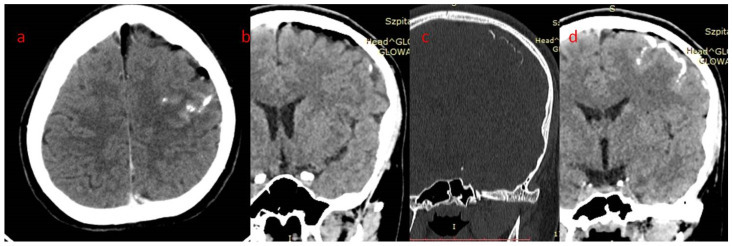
(a–d) Patient 2. Brain CT: gyral calcifications in left frontal lobe, coexisting subtle arachnoid space enlargement with small fat tissue amount. (a,b) Axial and reformatted coronal brain window—fat tissue; (c,d) left frontal calcifications: coronal reformatted (c) bone window and (d) brain window.
